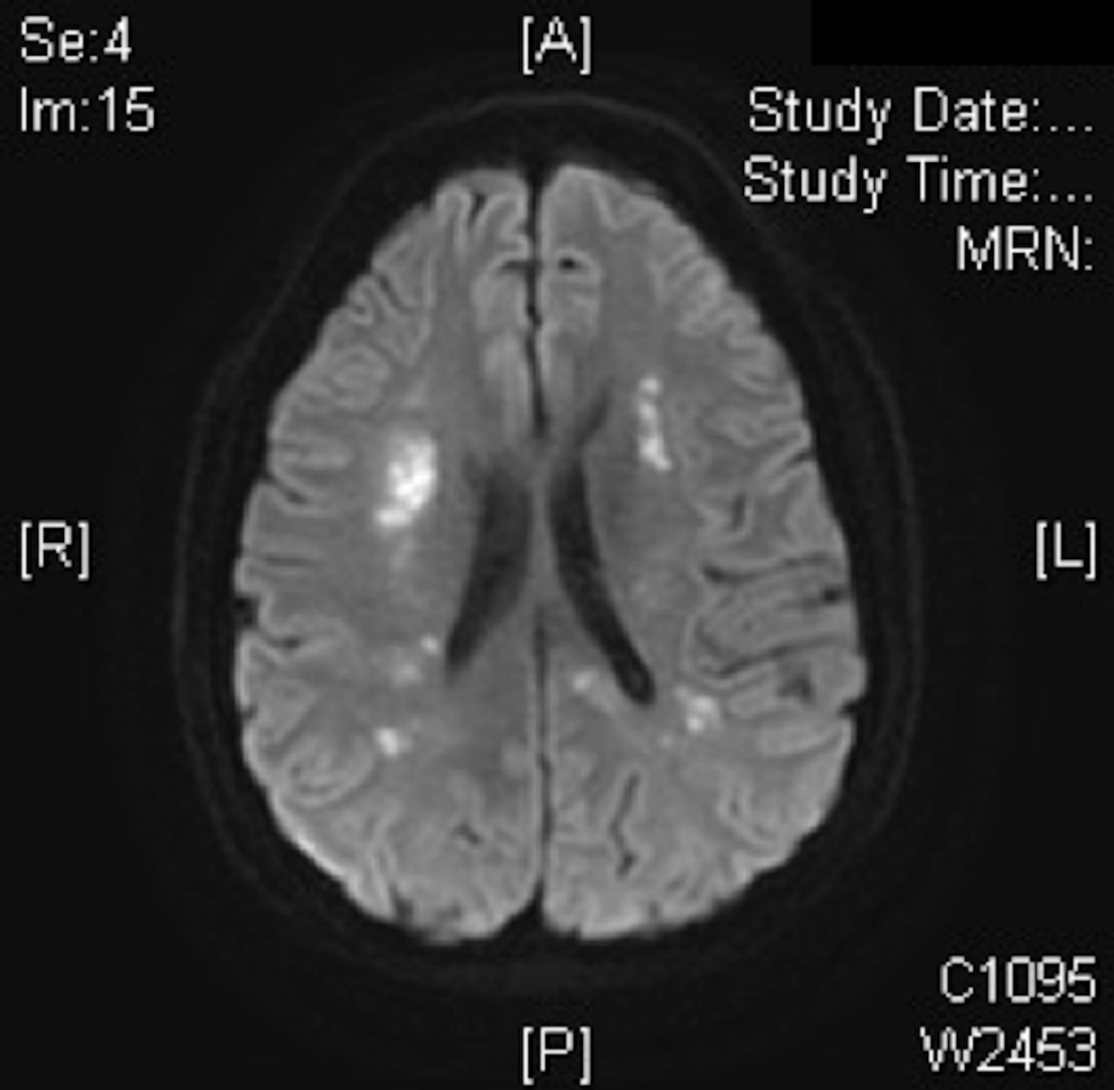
Hyperintensities on T2-weighted imaging, widespread confluent white matter hyperintensities
